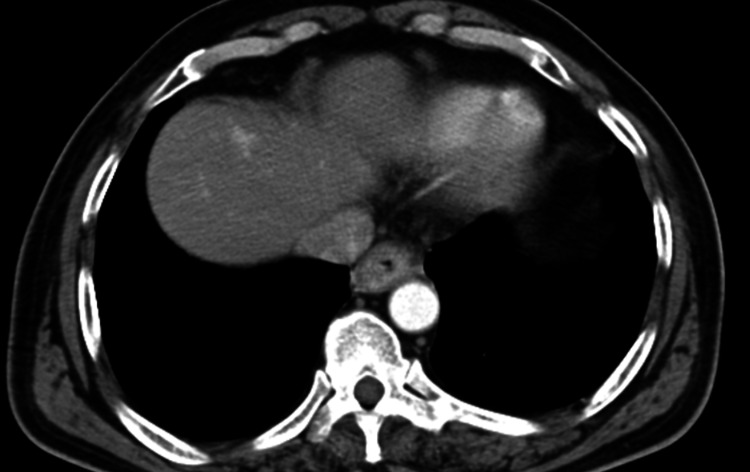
CT image of oesophageal carcinoma in the lower third region
